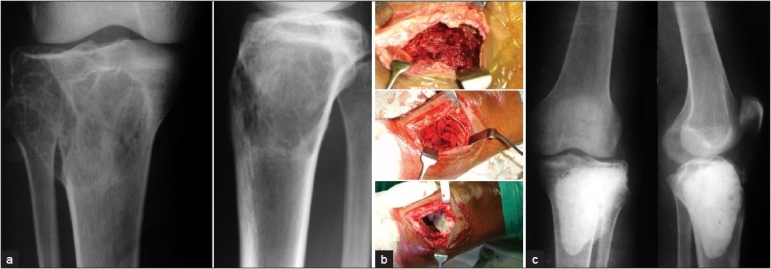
(a) X-ray (anteroposterior and lateral views) showing GCT of upper end of tibia, (b) intra-op photo showing (from above downwards) cavity after curettage, cancellous bone grafting, gel foam packing before cement application. (c) Post-operative X-ray (anteroposterior and lateral views) showing bone cement *in situ*
